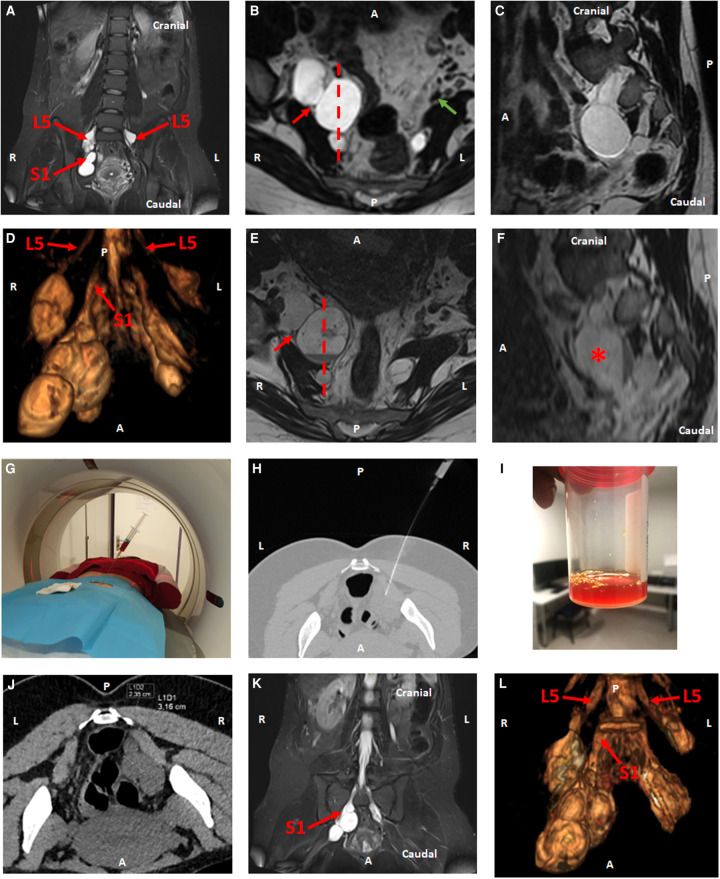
Presence of large bilateral sacral radicular cysts on MRI coronal (A), axial (B) and sagittal (C) T2-weighed sequences predominantly on the right L5 and S1, S2 and S3 roots (red arrows) and on the left L5 root (A). (C) correspond to dotted plane in (B). (B) Pelvic cystic formation of 36 × 29 mm on the right S1 nerve root (MRI axial view T2-weighed sequence), right (red arrow) and left (green arrow) sciatic nerves. (D) 3D reconstruction of the different cysts associated with the different nerve roots. Fluid-fluid level, on axial (E) and sagittal (F) T2-weighed sequences. (F) correspond to dotted plane in (E). Bleeding was noticed predominantly on the right L5 and S1 cysts (red asterisk) and appearing to sheath the distal portion of right S1 (red arrow). This intracystic hemorrhagic complication was probably due *post-partum* (G,H) Aspiration of a hemorrhagic liquid under pelvic-abdominal CT-scan guidance. (I) Fluid aspect. (J) Dimensions of this hemorrhagic cyst immediately after aspiration (CT-scan axial view): 31 × 25 mm. (K) MRI control 1 year after the procedure, with 3D reconstructions (L). Remodeling of the cyst was observed. A reduction of 7% of its initial volume was determined by volumetric analysis before and after the procedure, and the S1 root is better visualized. R = Right, L = Left, A = Anterior, P = Posterior.
